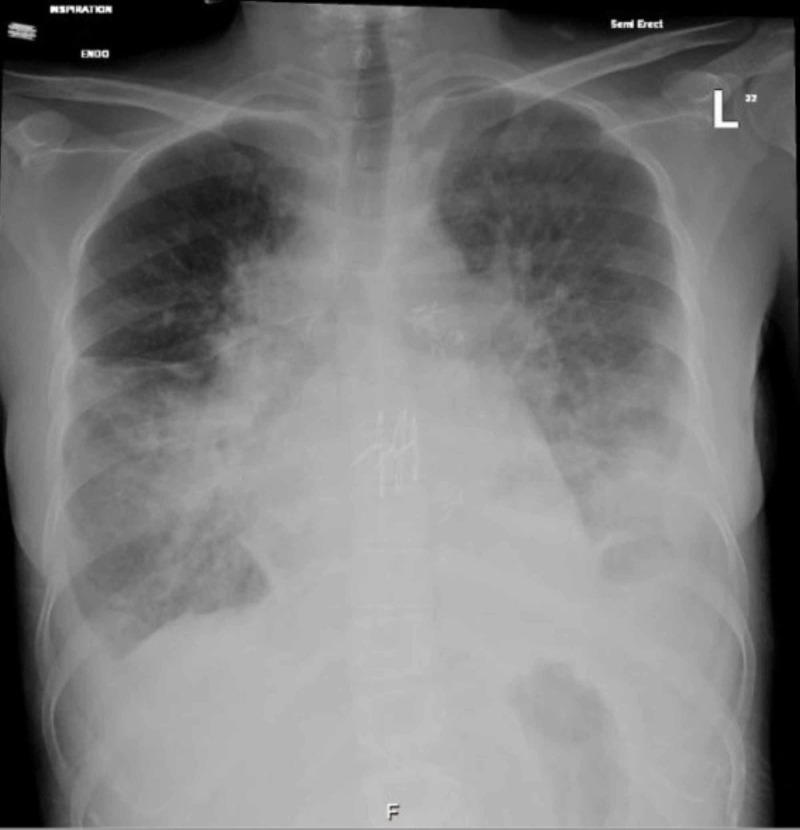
Patient’s initial chest X-ray shows bilateral lower air space and perihilar opacity, bilateral pleural effusion.
